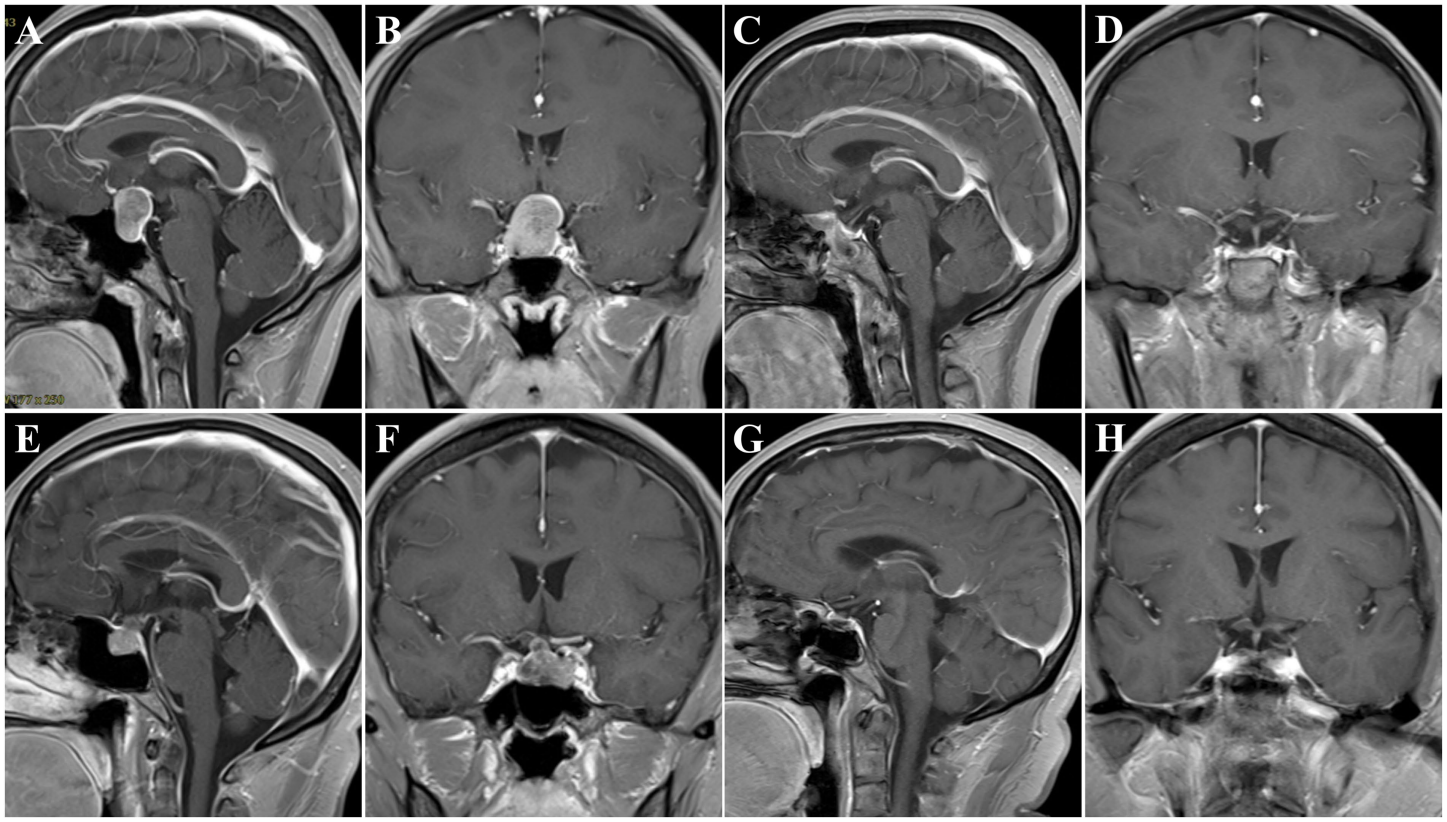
Preoperative and postoperative T1 MR images. (A–D) Patient No. 2, preoperative sagittal (A) and coronal (B) images showing a PitNET, Knosp grade 2. Three-month postoperative sagittal (C) and coronal (D) images showing GTR. (E–H) Patient No. 8, preoperative sagittal (E) and coronal (F) images showing a PitNET, Knosp grade 0. Three-month postoperative sagittal (C) and coronal (D) images showing GTR.
